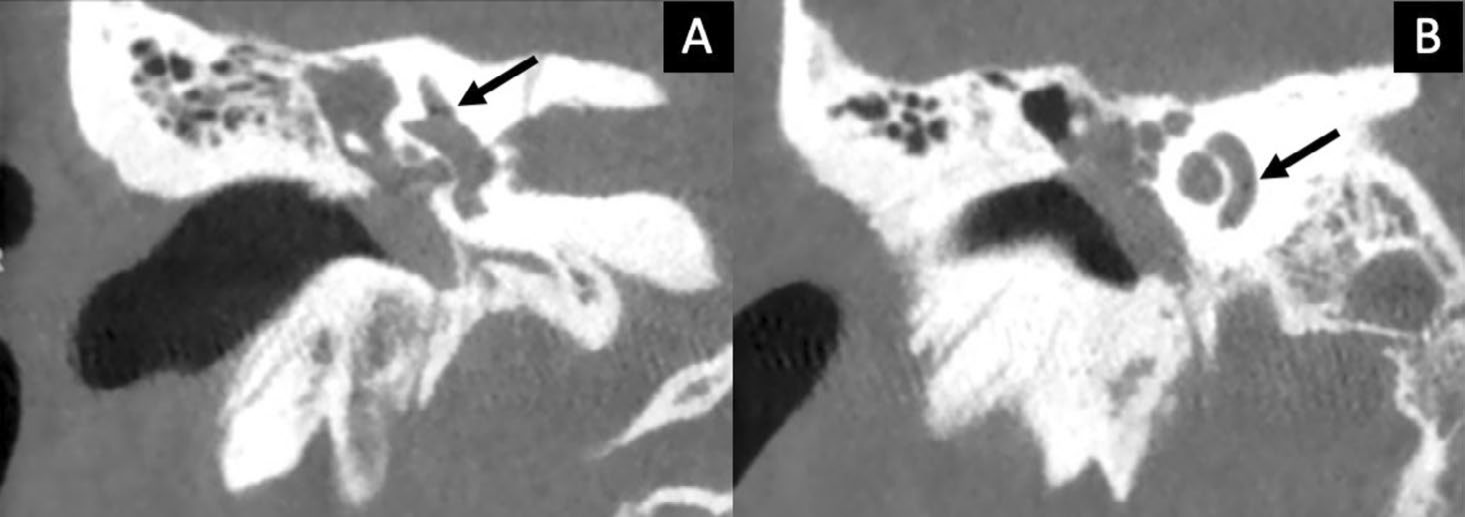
(A) CT: Transverse petrous bone fracture with air in the superior semicircular canal. (B) Air bubbles in the cochlear basal turn.
